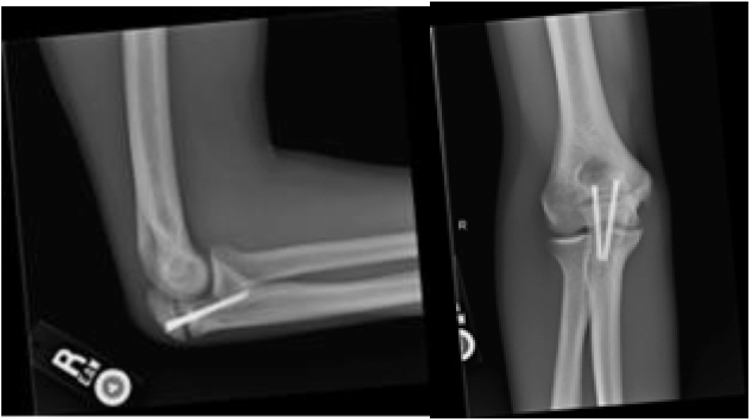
4-week postoperative plain films: persistent transverse olecranon fracture nonunion with two well-aligned headless compression screws and resorption of bone graft.
